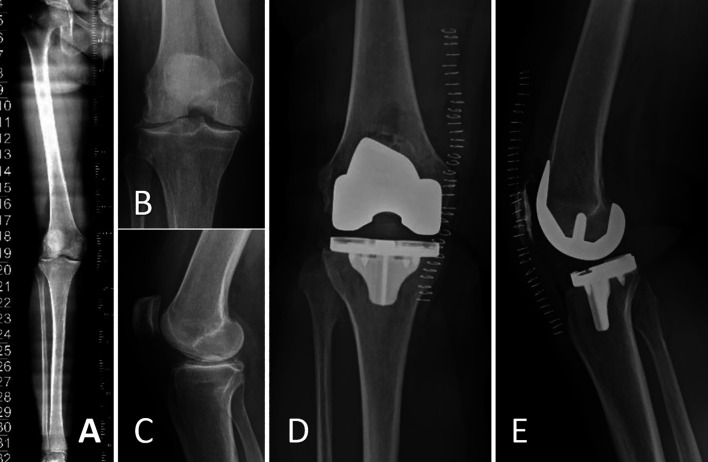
Pre- and post-operative X-ray views of a valgus knee with osteoarthritis treated with image-based total knee arthroplasty under the principles of Functional knee positioning. (A) Preoperative long axis weight-bearing Anteroposterior (AP) view, (B) AP view of the knee, (C) Lateral view of the knee, (D and E) AP and lateral postoperative X-ray views showing the implantation of a cementless femoral and tibial component (Triathlon, Stryker, MI, USA).
